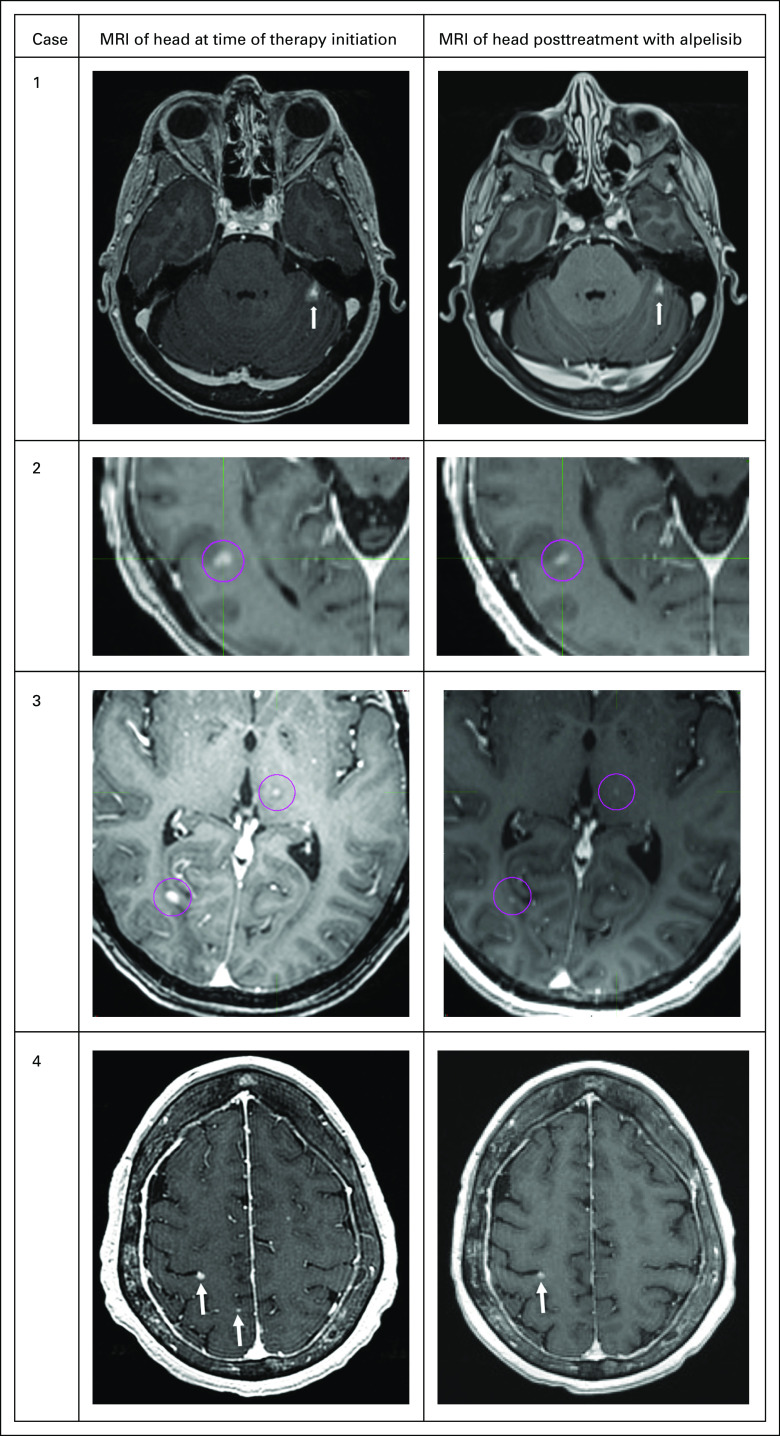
CNS response measured by magnetic resonance imaging (MRI) of the brain. Pink circles and white arrows represent metastatic disease from breast cancer.
